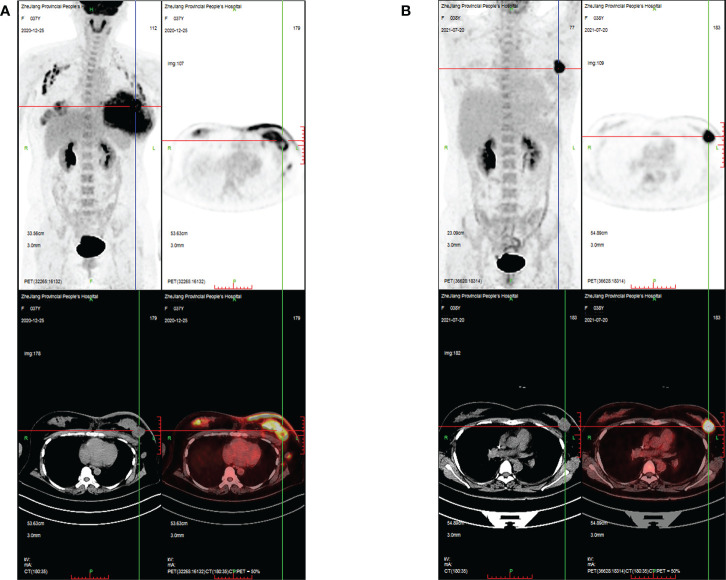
PET/CT images evaluating the changes in breast masses and metastatic lymph nodes during treatment in case 1. (A) PET/CT images showing intense FDG uptake in the bilateral breast, bilateral axilla, left clavicular area, and left upper mediastinum at the time of initial diagnosis; (B) PET/CT images showing that areas of high FDG uptake in the breast and lymph nodes were significantly reduced after 10 cycles of chemotherapy.
